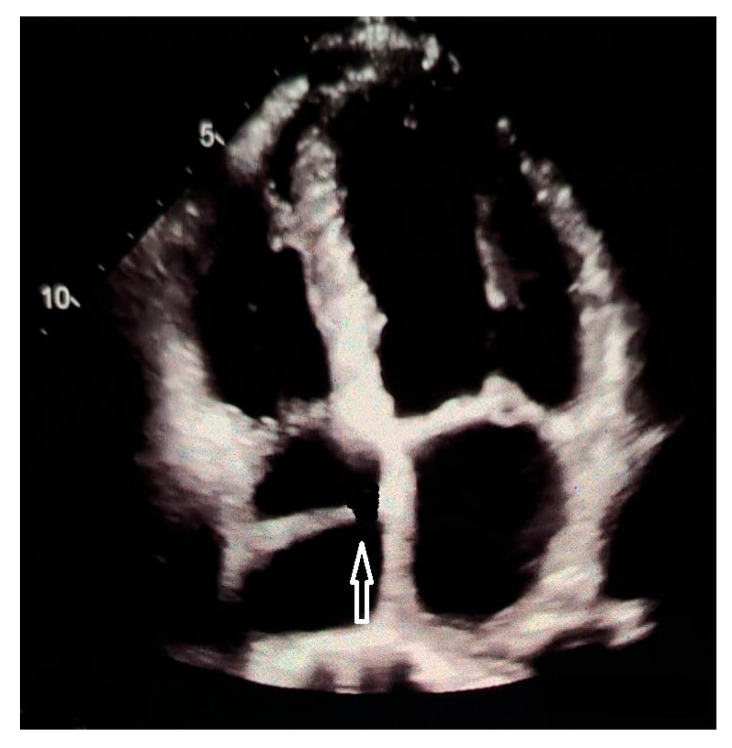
Transthoracic echocardiogram (4 chamber-view) showing a slightly mobile, linear, echogenic structure not reaching the interatrial septum (prominent Eustachian valve, white arrow).
